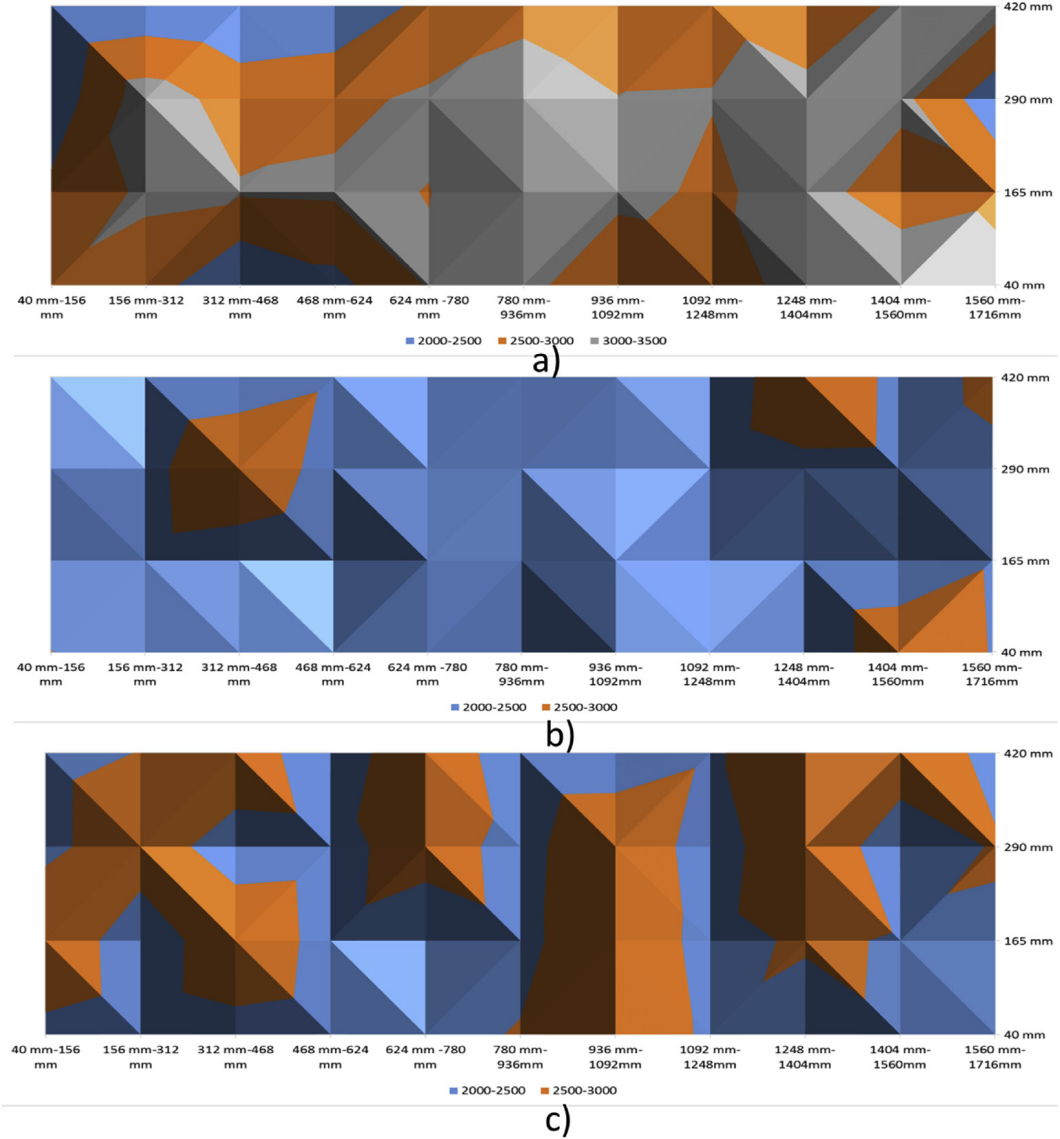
Ultrasonic Pulse Velocity-Control Slabs - a) 100 mm, b)150 mm, and c) 200 mm.
